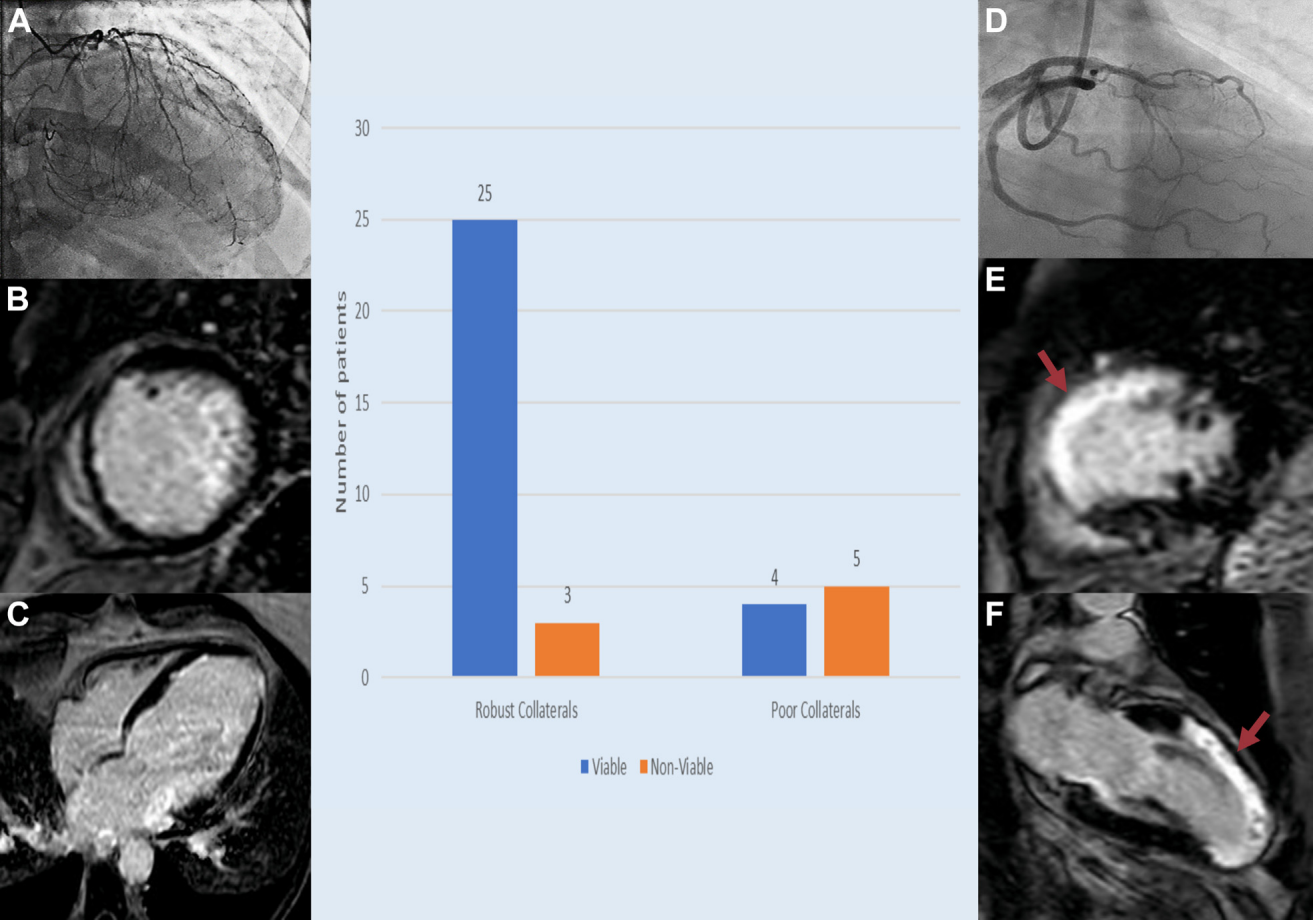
Graphical presentation of degree of angiographic collaterals to the cardiac magnetic resonance imaging (MRI)-derived viability of the myocardium in patients with chronic total occlusion. (A) Robust collaterals of RCA and LAD with concomitant viability with <50% late gadolinium enhancement in the respective territories on cardiac MRI in short axis (B) and long axis (C). (D) Poor collaterals of LAD with concomitant nonviability with >50% late gadolinium enhancement (red arrows) in the LAD territories on cardiac MRI in short axis (E) and long axis (F) Created in BioRender. S. Sohal (2025). https://BioRender.com/s95k830.
